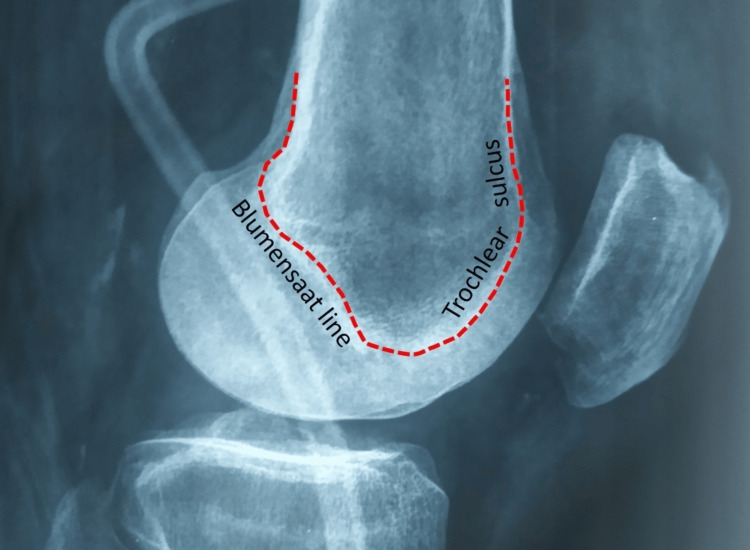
Assessment of TD on the true lateral plain X-ray of the knee The red line represents the intercondylar line of the distal femur. The anterior part of the intercondylar line is cartilaginous and represents the trochlear sulcus. The posterior continuation of the intercondylar line is osseous and represents the roof of the intercondylar notch. The posterior part of the intercondylar line is known as the Blumensaat line. Figure created by Panagiotis Samelis.
